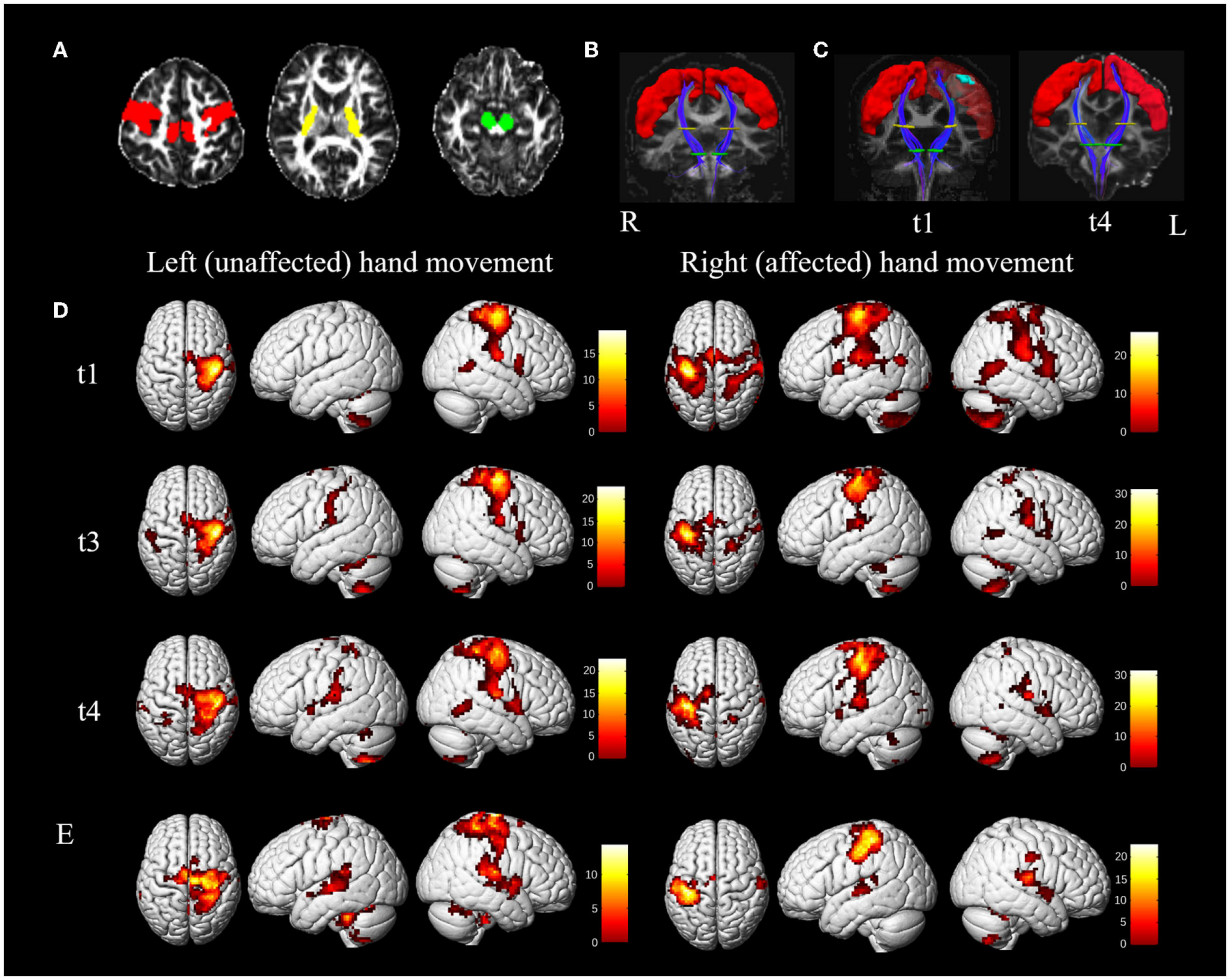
MRI results. (A–C), diffusion tensor tractography of the corticospinal tract (CST). (A) Regions of interest (ROI) for reconstructing the CST are located at the precentral gyrus (red color, obtained from the AAL90 template), the posterior limb of the internal capsule (yellow color), and the cerebral peduncle (green color) on the fractional anisotropic (FA) map. (B) A coronal view of the bilateral CSTs (blue color) from the healthy control. The red, yellow, and green areas represent the ROI of the precentral gyrus, the posterior limb of the internal capsule, and the cerebral peduncle. (C) A coronal view of bilateral CSTs from the patient at t1 (5 weeks) and t4 (45 weeks). On the left of the C map (t1), the cyan region is the lesion registered from T1 space to FA space. (D,E) activation during passive unaffected (left) and affected (right) hand movements of the patient and healthy control. (D) Activation of passive movement of the patient at t1, t3 (12 weeks), and t4; (E) activation of passive movement of healthy control. Color bar = *t*-value. The left side indicates the left hemisphere. L, left; R, right.
